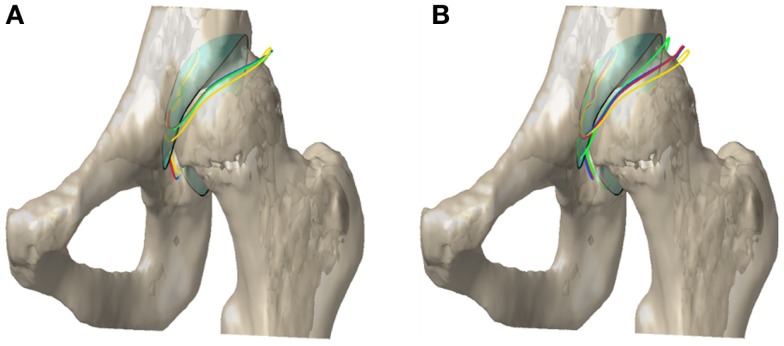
Acetabular contact surface outline in the original and optimal alignment for (A) linear and (B) non-linear DEA. The colors red, blue, green, and yellow correspond to uniform, population-based (p-b) normal, p-b dysplastic, and CT-based cartilage thickness models, respectively. The cyan surface with the black outline represents the original alignment of the contact surface.
